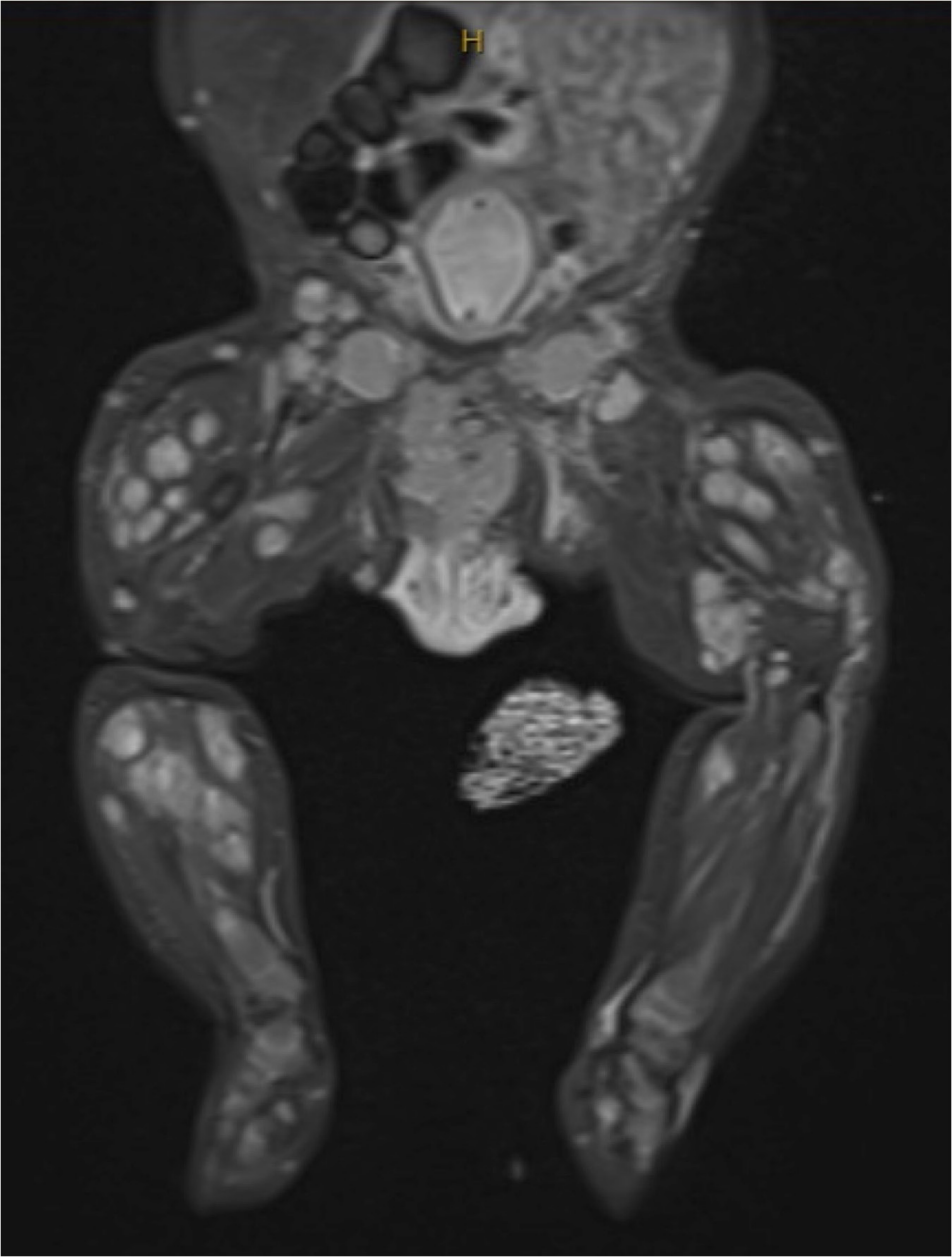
MRI imaging showing disseminated disease throughout the legs and abdomen
